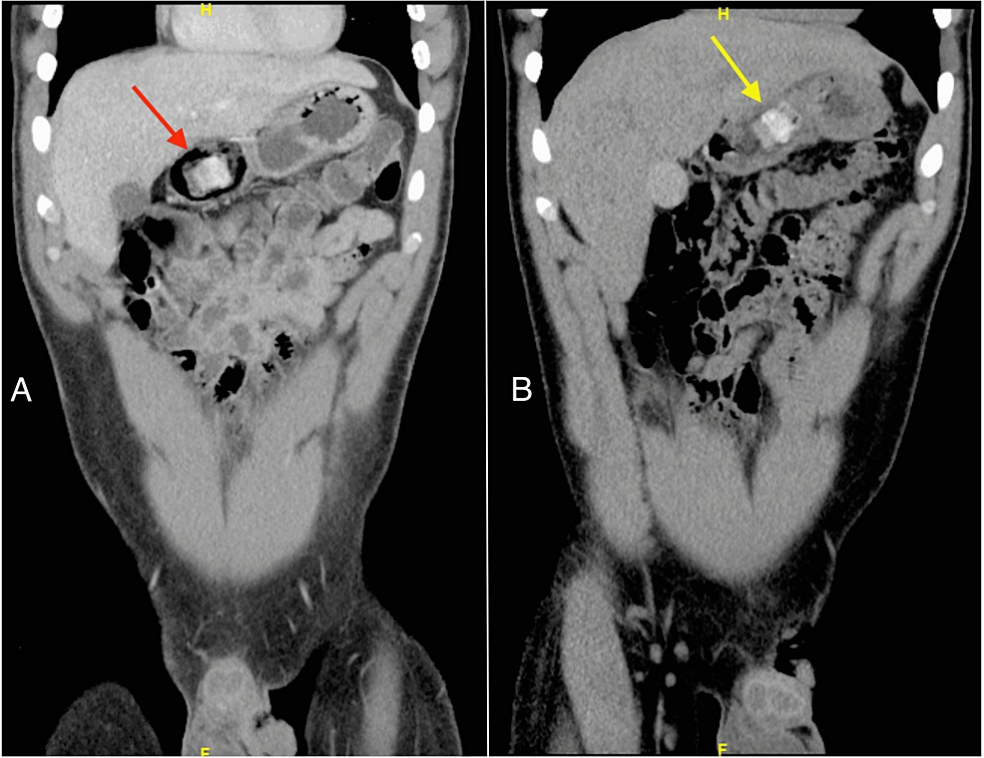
Computed tomography of the abdomen: (A) at admission, showing one of the packages in the gastric antrum (red arrow); (B) after 48 hours, showing the persistence of heroin bags in the gastric antrum with minimal movement (yellow arrow).
